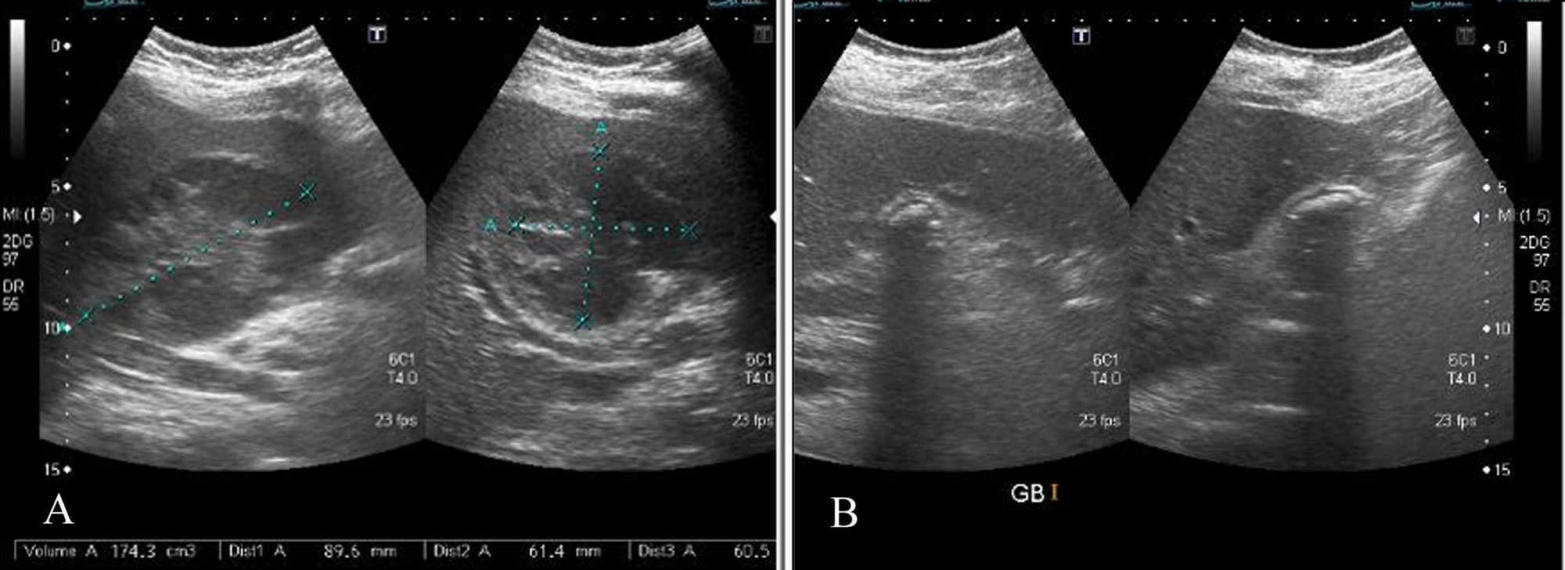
Ultrasound images A) Liver abscess in segment IV, with a volume of approximately 175 cc. B) Contracted gallbladder containing numerous calculi giving wall echo sign.
